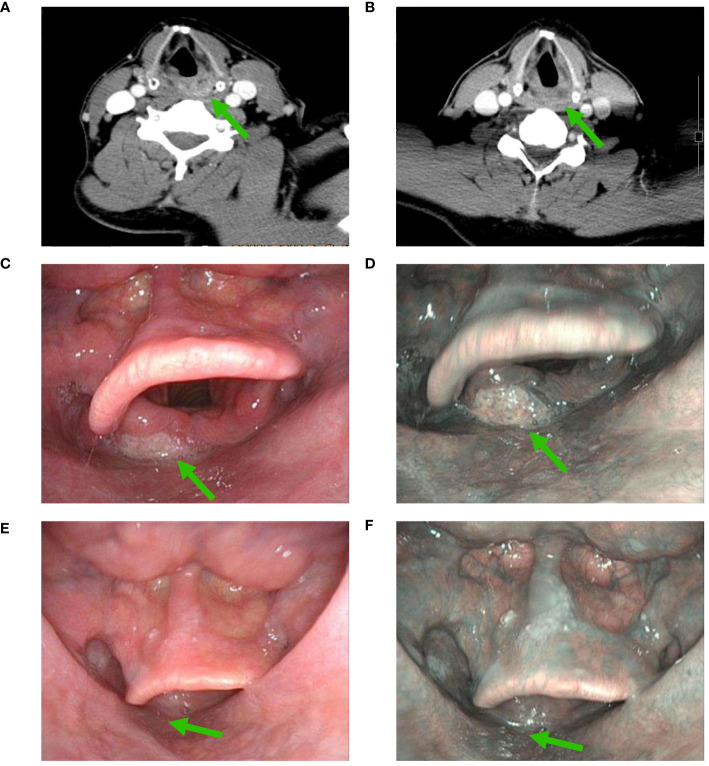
Changes in a patient with significant downgrading on NBI assessment after NAC and ICI (A), CT scan before NAC and ICI; (B), partial response using CT assessment after NAC and ICI; (C), white light imaging assessment before NAC and ICI; (D), NBI assessment before NAC and ICI; (E), white light imaging assessment after NAC and ICI; (F), a significant downgrading on NBI assessment after NAC and ICI (CT, computed tomography; NAC, neoadjuvant chemotherapy; ICI, immune checkpoint inhibitor; NBI, narrow band imaging) (The green arrow refers to the primary hypopharyngeal lesion).
